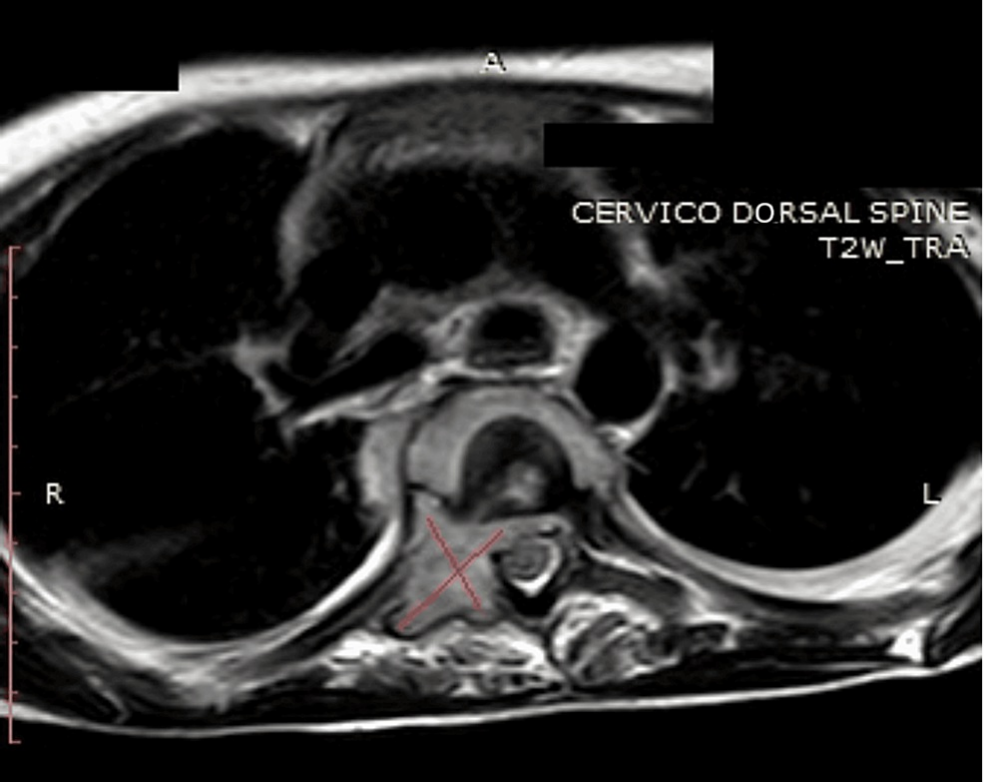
MRI cervical-dorsal spine T2W shows an extension to the paraspinal muscle.
